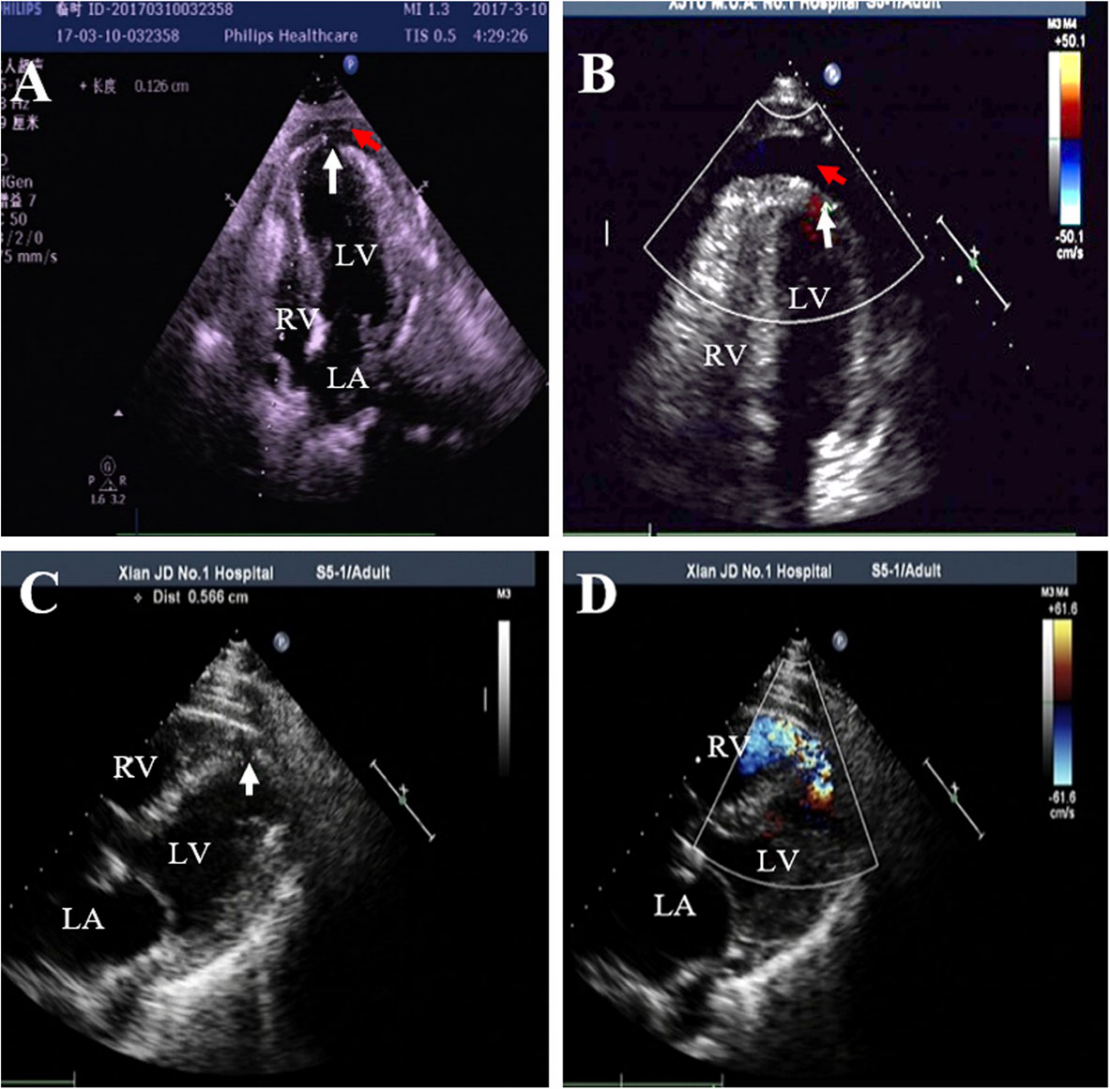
Representative transthoracic echocardiographic images from patients with cardiac rupture. a 2-D image revealed discontinuity of the left ventricular (LV) wall (white arrow) and pericardial effusion (red arrow). b Color Doppler image demonstrated shunting of blood flow from the LV to the pericardium (white arrow) and presence of pericardial effusion (red arrow). c ventricular septum discontinuity (white arrow) in 2-D image. d shunting of blood from the LV to the right ventricle (RV, red arrow) in Color Doppler image; LA = left atrium; RA = right atrium
